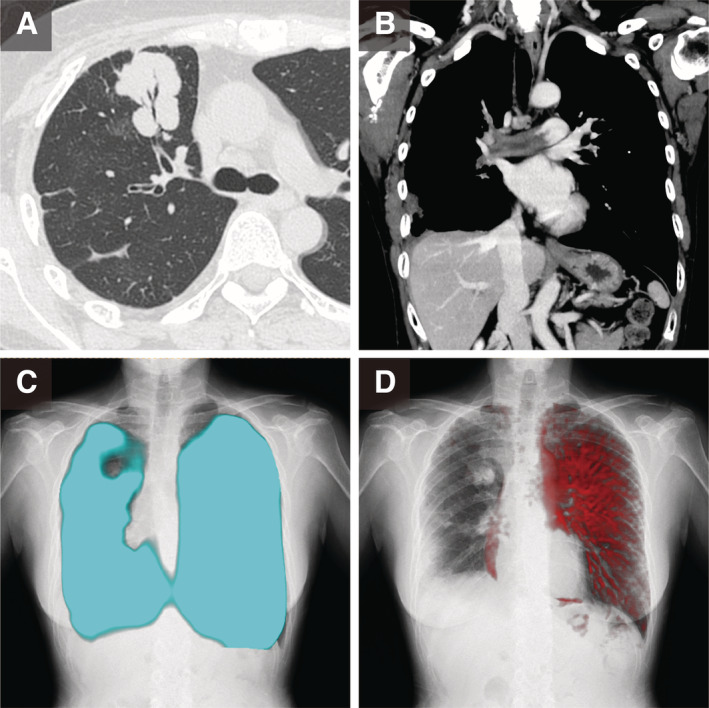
Chest imaging. (A) Chest computed tomography (CT) (lung window). The air bronchogram indicated three nodules (range: 14–26 mm) and interstitial thickening in the right upper lung lobe (S^3^). (B) Coronal contrast chest CT (mediastinal window). Severe stenosis in the right pulmonary artery was due to pulmonary arterial wall thickening and a low‐attenuated lesion in the right pulmonary artery. (C) A chest digital dynamic radiography (DDR) ventilation image showing pulmonary air filling during deep breathing for 15 sec, indicating almost normal bilateral lung ventilation dynamics. (D) A chest DDR perfusion image showing the blood supply while breath‐holding for 6 sec as slight changes in pixel value even without the use of contrast media. Severely decreased perfusion in the entire right lung can be observed.
